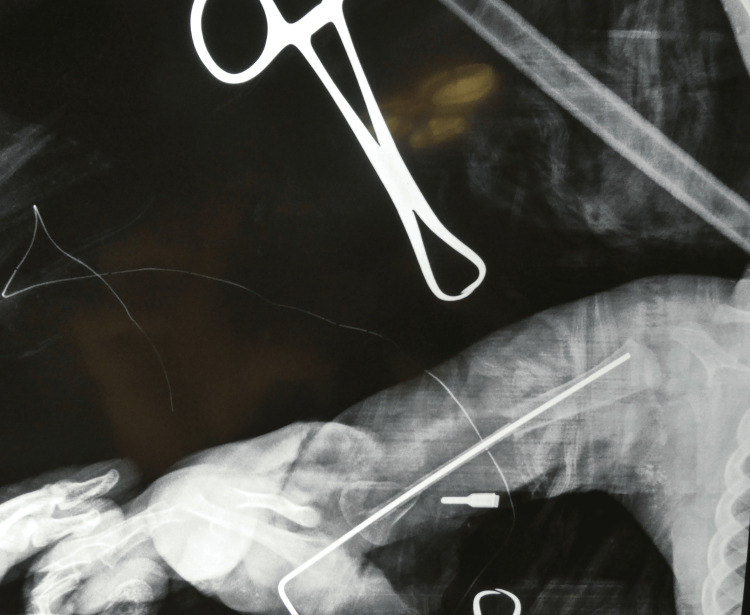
Immediate postoperative radiograph of humeral fixation with an intramedullary K-wire
